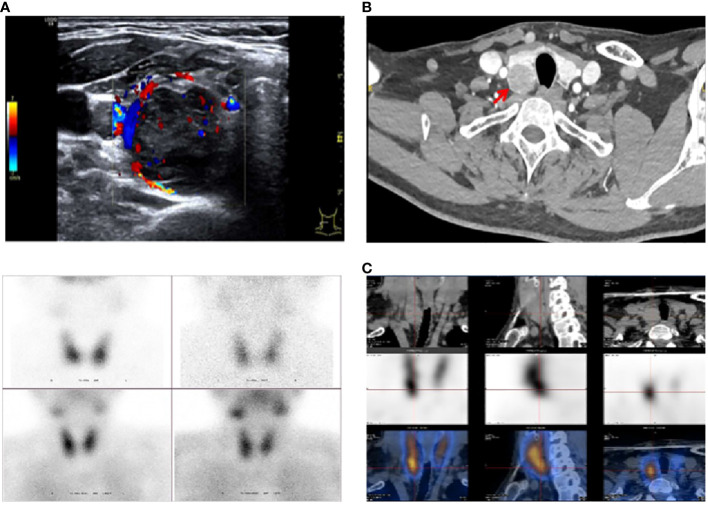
Imaging studies in the proband. (A) Parathyroid ultrasound: Dorsal aspect of the inferior pole of the suitable lobe: 3.1×2.4×1.9 cm. (B) Neck CT enhancement: Dorsal aspect of the inferior pole of the right lobe: Parathyroid origin? (C) Parathyroid ECT (99TcmO4¯MIBI): Right inferior parathyroid hyperfunctioning lesion: 2.2×2.3×2.9 cm.
